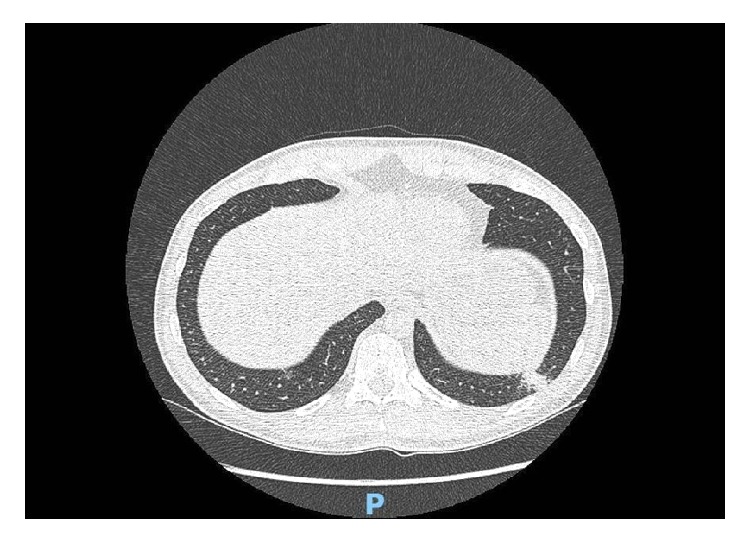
CT of chest showing lower left lobe nodule (Case 2).
